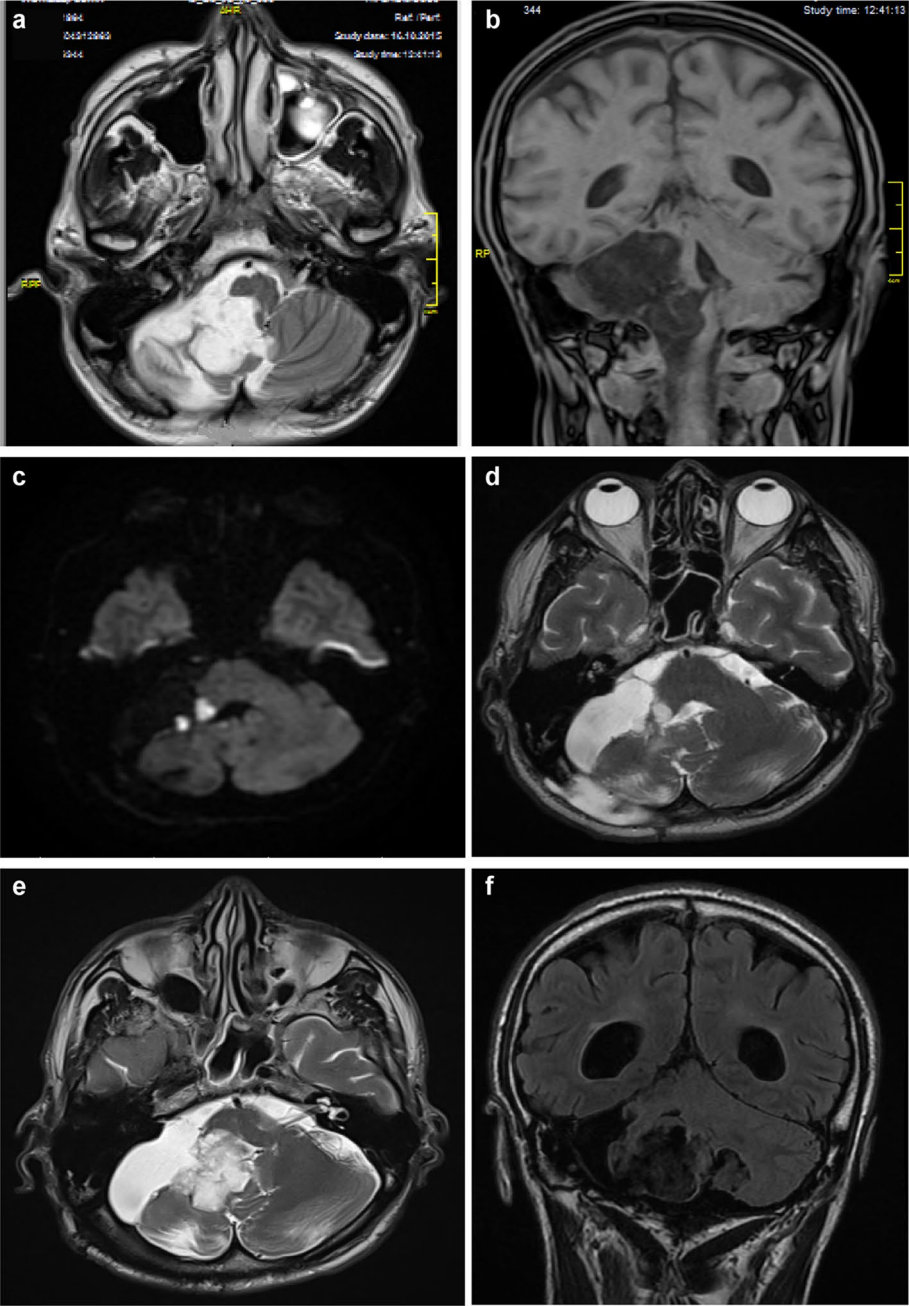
Patient 2, recurrent cerebellopontine angle epidermoid cyst, preoperative MRI (performed in 2015). Images (a) and (b) T2 and T1 sequences, respectively, revealed an epidermoid cyst located in the right cerebellopontine angle. Three-month follow-up MRI (images c and d—DWI sequence and T2 sequences, respectively) demonstrated a subtotal resection with a small remnant deeply located in the right middle cerebellar peduncle. The patient was asymptomatic, so he was monitored periodically with MRI imaging at 6-month intervals. The last MRI performed in 2022 demonstrated a large recurrent tumoral mass, but the patient was neurologically stable and asked for delayed reintervention (image e—T2 sequence and image f—T1 sequence).
